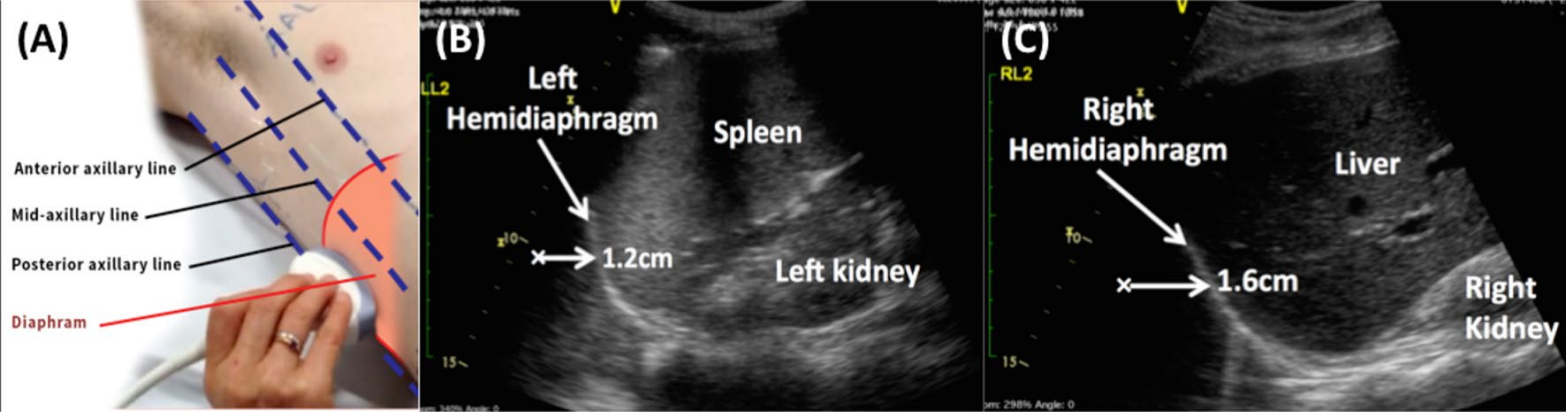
Ultrasound technique and images of the diaphragm. A The location of probe placement on the chest wall for assessment of diaphragm movement. The probe is placed laterally and perpendicularly on the lateral chest wall on the lower intercostal spaces between mid- and posterior axillary line. B, C The ultrasound images of the left and the right hemidiaphragm and the measurement of the diaphragmatic excursion from end of end inspiration to end expiration
